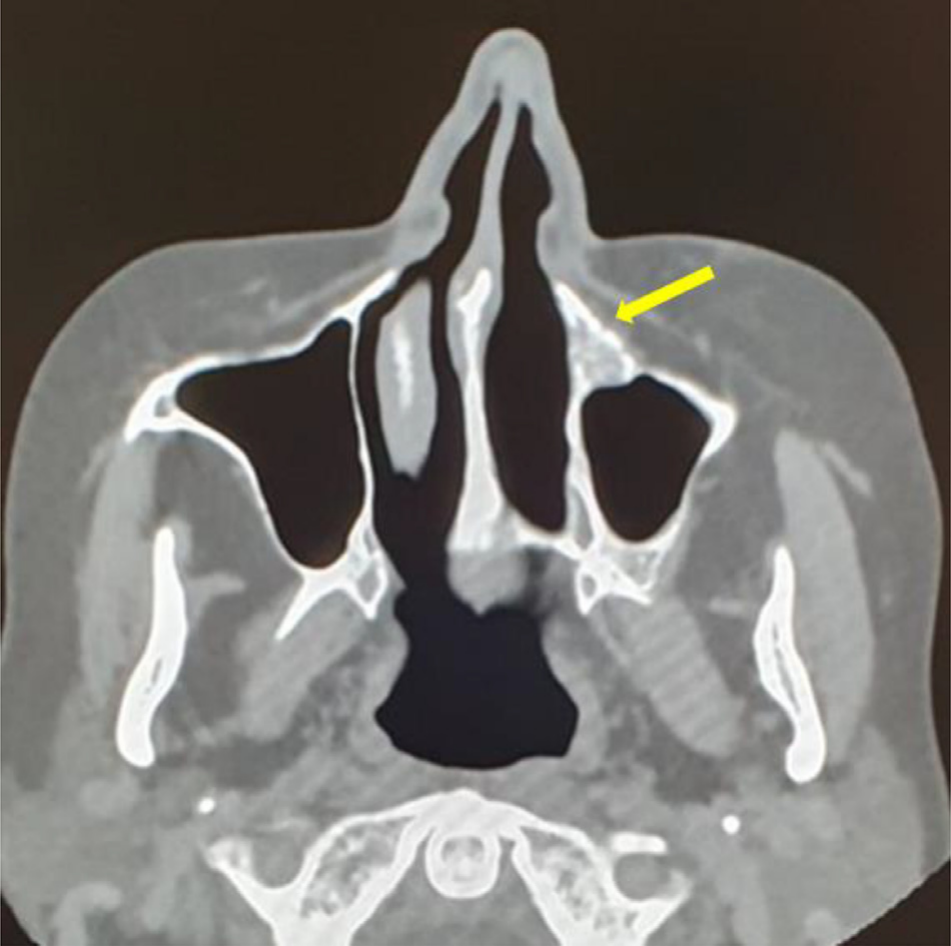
Follow-up CT showed reduction in lesion size. Presence of small residual neoplastic tissue at the level of the antero-medial region of the left maxillary sinus was detected, evidence of therapeutic efficacy.
